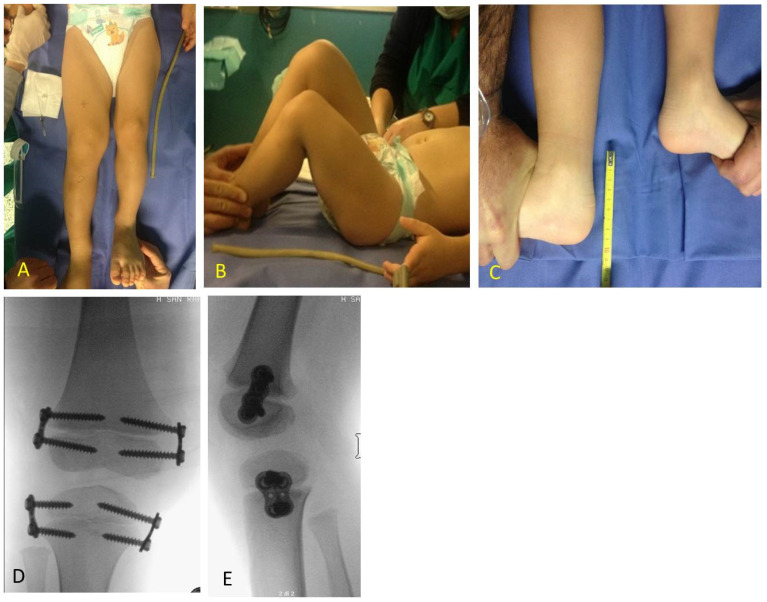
BWS male child with LO (lateralized overgrowth) right and severe LLD (leg length discrepancy): (A) clinical aspect showing both femur and tibia length discrepancy; (B) femur and tibia length discrepancy is clearly visible from lateral with flexed hips and knees; (C) LLD of 10 cm is present; (D,E) PTE (temporary proximal tibia epiphysiodesis) and DFE (distal femur epiphysiodesis) were performed in one step at same time at age of 2.11 years, X-ray in antero-posterior projection showing position of guided growth system with 8-plates straddles distal femoral physis and proximal tibial physis.
